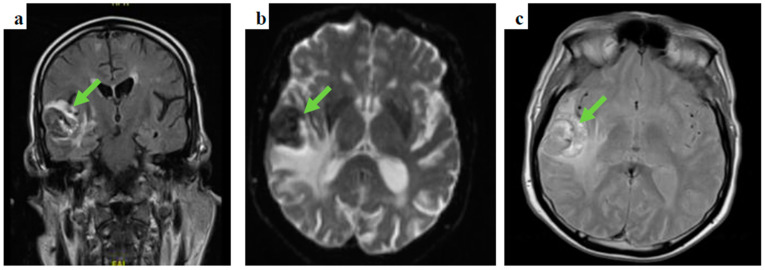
Gadolinium-enhanced brain MRI images: (a) coronal, fluid-attenuated inversion recovery (FLAIR) sequence showing a right temporal lobe lesion, (b) an axial, diffusion-weighted image (DWI), b1000 showing the temporal lesion surrounded by vasogenic oedema, and (c) an axial, T2-weighted imaging showing a high intensity lesion in keeping with the presence of either subacute stage blood products or melanin.
